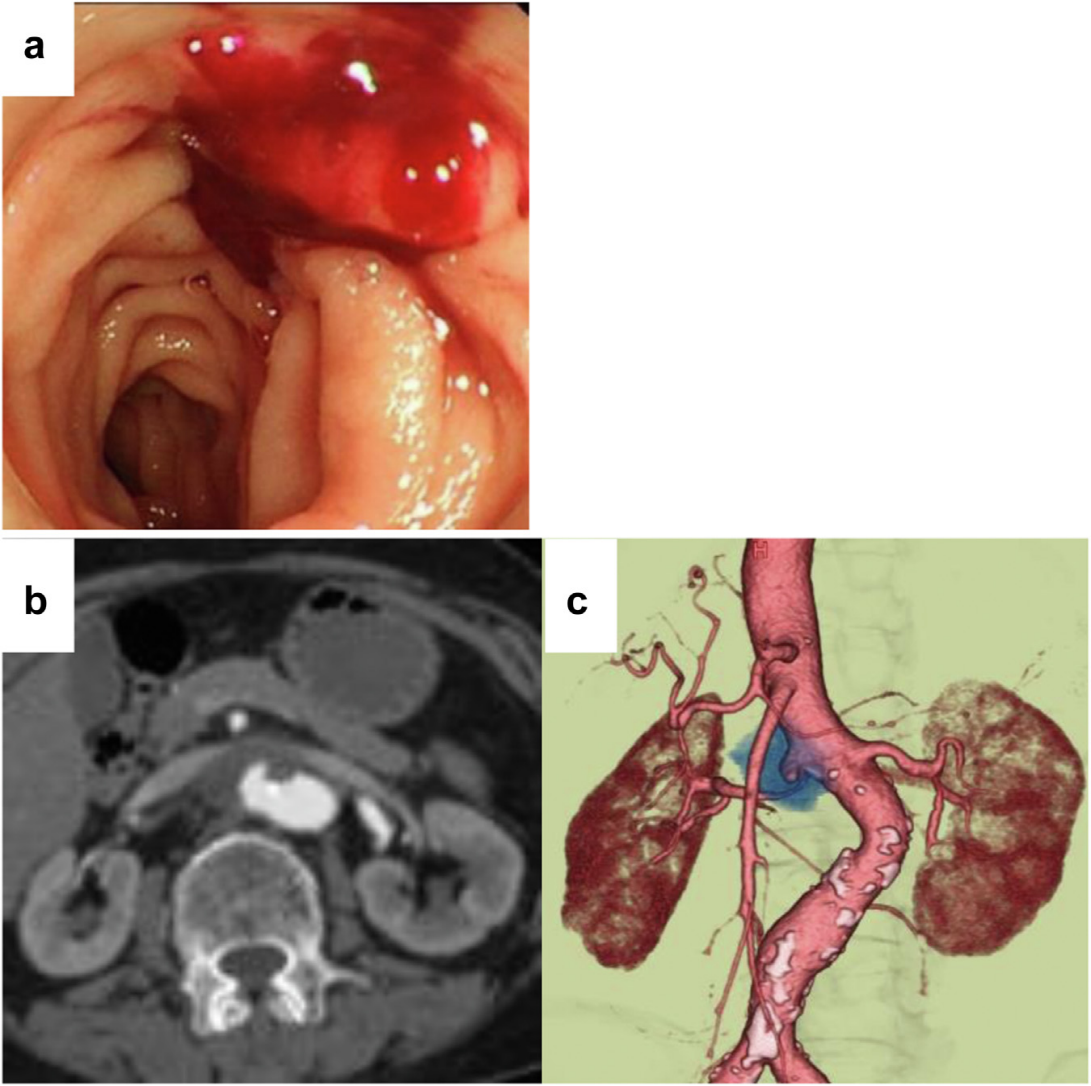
Preoperative enhanced abdominal computed tomography (CT) scan and endoscopy. a, Endoscopy demonstrated the fresh clot in the third portion of duodenum. There was no active bleeding. b, Axial view of the saccular aneurysm just above the right renal artery. c, Three-dimensional CT scan of the aneurysm oppressed the right renal artery.
